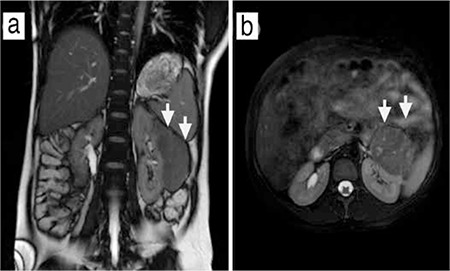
Magnetic resonance imaging of the renal tumor a) coronal section, b) axial section (arrow indicating the tumor)
